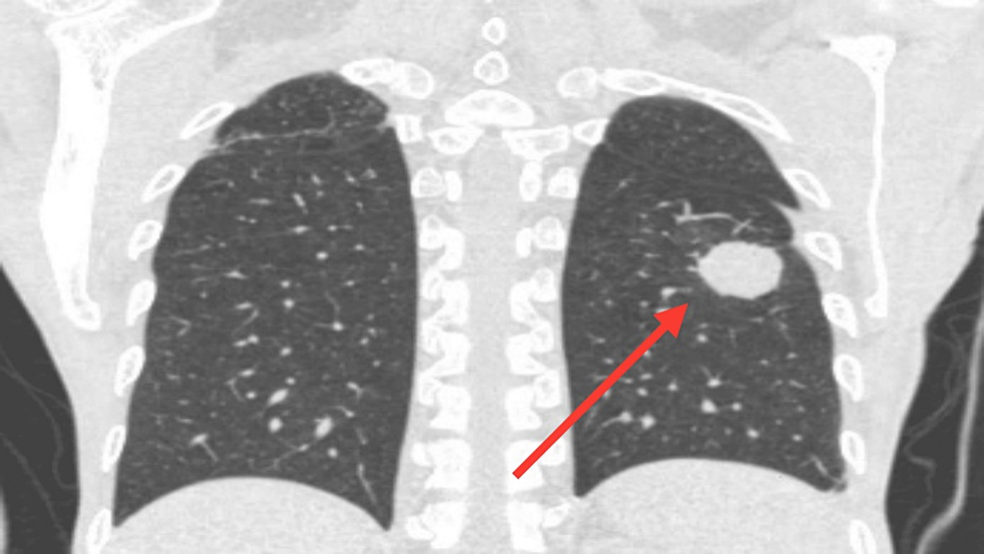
Solitary pulmonary nodule in the left lower lobe in the superior segment as seen in the current coronal section of CT thorax figure with red arrow.
